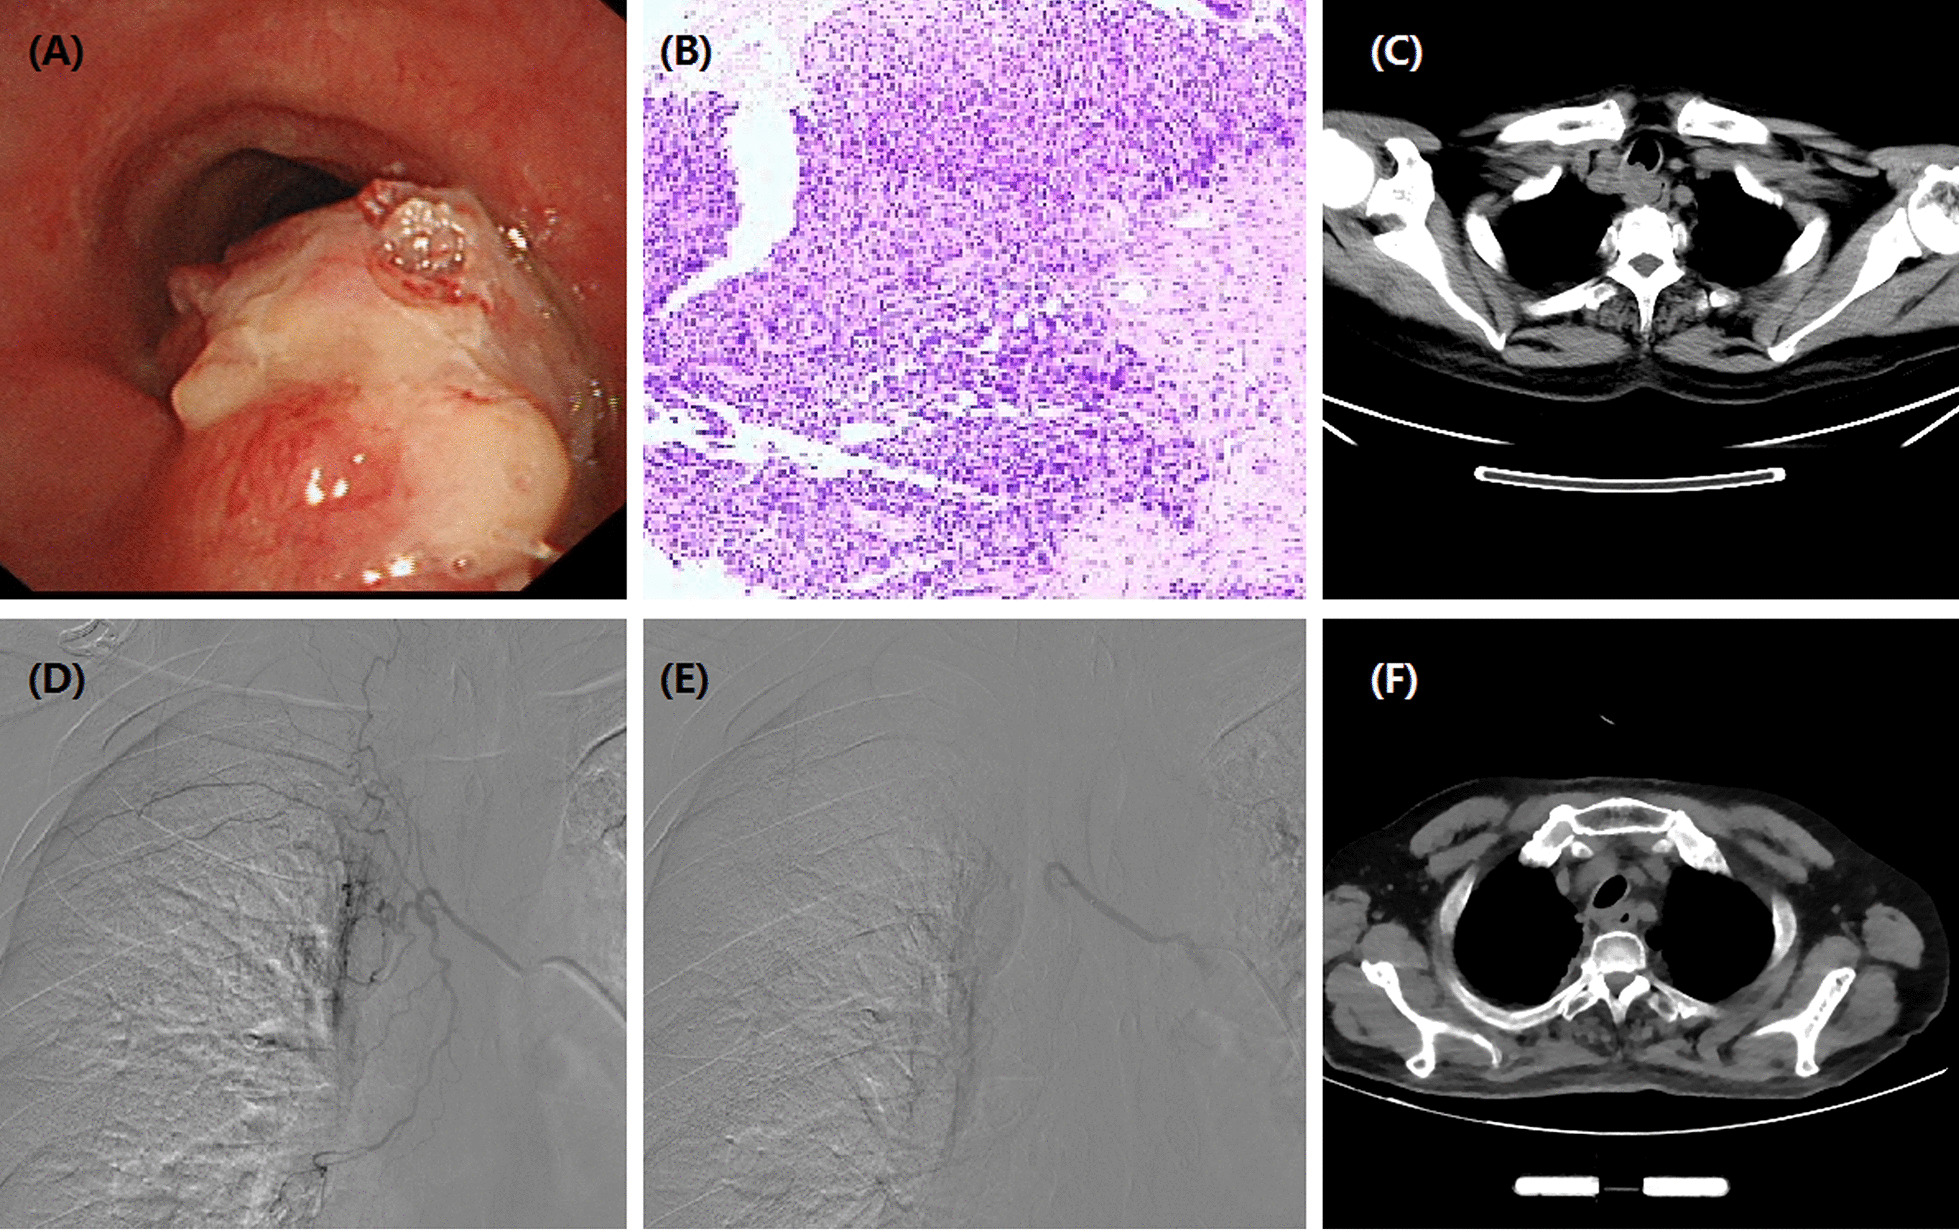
Images of a 66-year-old man with esophageal cancer in upper and middle esophagus. aBrancheoscopy shows esophageal tumor ingrowths into tracheal wall. bPathological diagnosis of squamous cell carcinoma. cCT scan shows an obvious thickening of esophageal wall and invasion into tracheal wall. dThe right bronchial artery is super-selectively catheterized by a microcatheter. eTumor-feeding artery was embolized using doxorubicin loading-CalliSpheres beads. fAfter 1 month, CT scan shows a stable mass in the esophageal body
